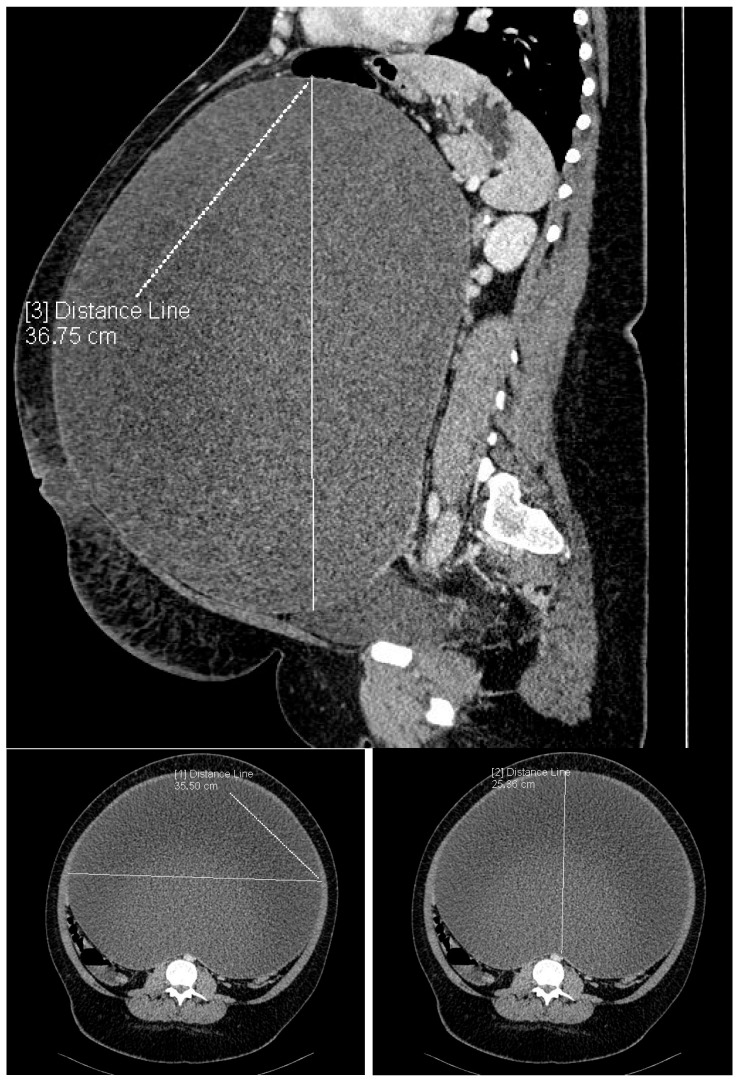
The CT scan of the abdomen and pelvis: measurements of the cystic mass.
